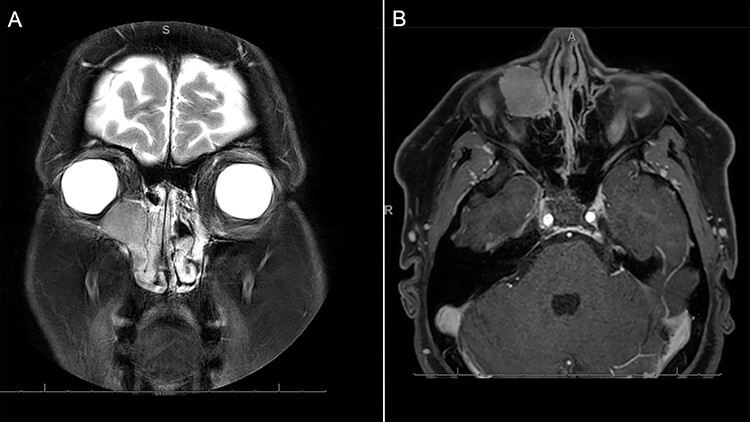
MRI demonstrating (A) coronal T2 demonstrating a homogenous, markedly hyperintense lesion of the right medial orbit, extending into the lacrimal duct. The contrast enhanced axial T1-weighted imaging demonstrates homogenous enhancement of the lesion with contrast.
